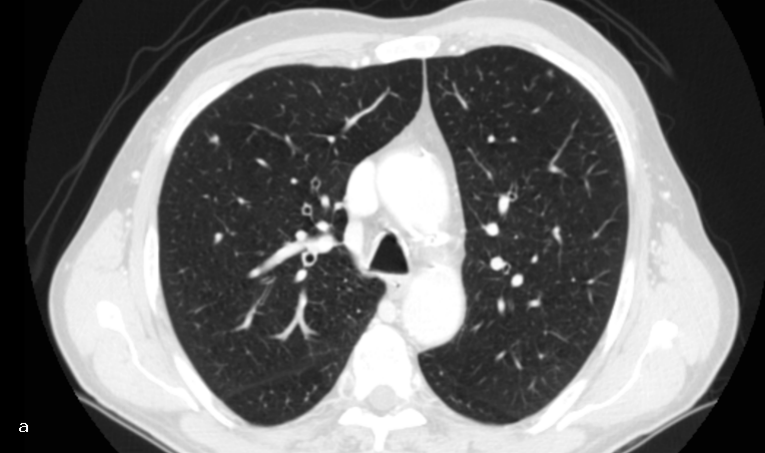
Lung chronic inflammation. Patient #8. Lung chronic inflammation in the right upper lobe. (a) CT recognized the lung nodule as malignant (FP CT) and (b) PET/CT did not reveal increased FDG in relation to the size of the nodule (TP PET/CT).
